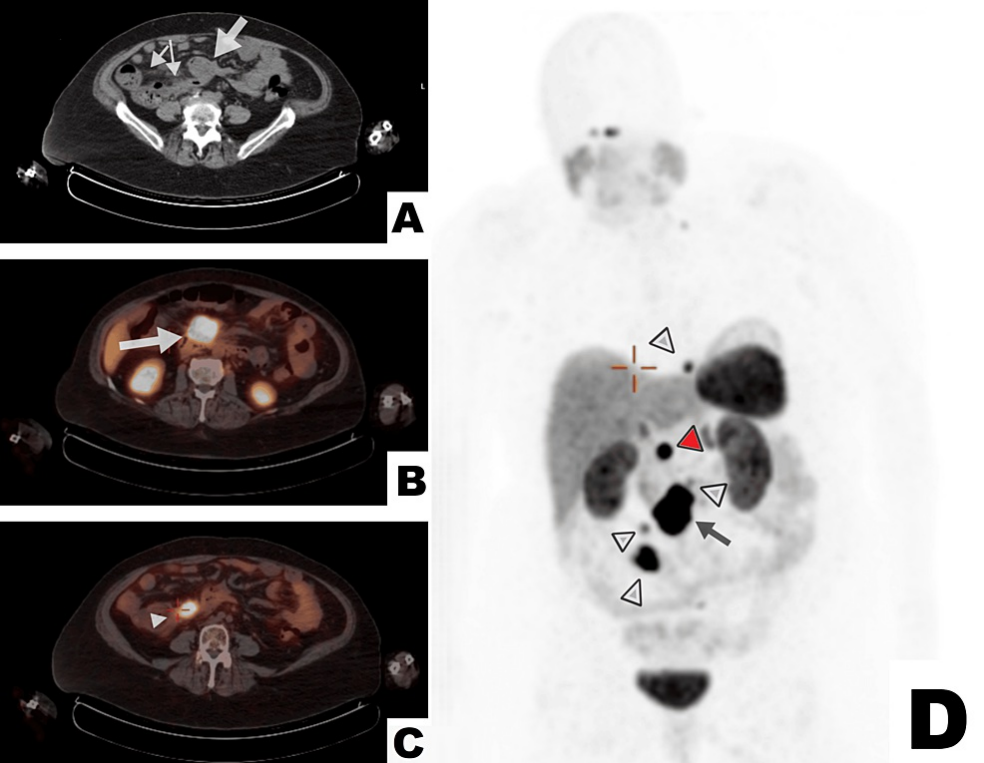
Imaging findings suggesting the diagnosis of a neuroendocrine tumor. A: A transverse CT section of the lower abdomen showing an irregular mass just anterior to the ileum (thick arrow) and thickening of the cecal and ileal walls (thinner arrows). B: Increased uptake of ^64^Cu-DOTATATE (arrow) by the malignant mass on positron emission tomography (PET) scan. C: Increased ^64^Cu-DOTATATE uptake (arrowhead) by a sizeable secondary lesion inferolateral to the primary on PET scan. D: Increased ^64^Cu-DOTATATE uptake in the primary lesion (arrow) and metastases (light arrowheads) on maximum intensity projection image of PET scan, coronal section. Note: The increased radiotracer uptake indicated by the red arrowhead represents the normal functioning of the uncinate process of the pancreas. The unlabeled opacities of the kidneys, liver, spleen, bladder, adrenal, salivary, and pituitary glands also represent the physiologic uptake.
